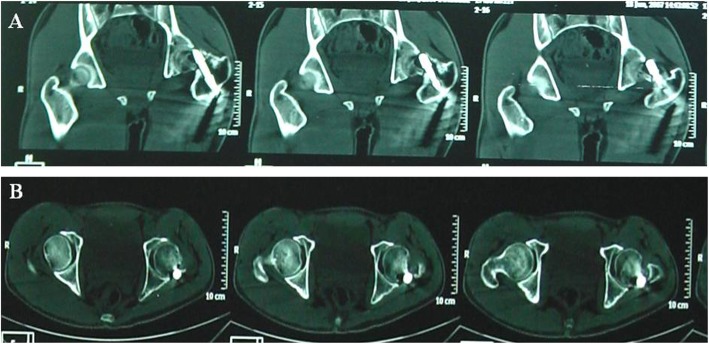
Patient, male, 72 years old, left femoral intertrochanteric fracture, 109 days after DHS fixation. CT scan showed left hip varus deformity and limb shortening. DNS screw appeared to cut and nail tail protruded femoral head. a hip CT coronal position, b hip joint CT level
